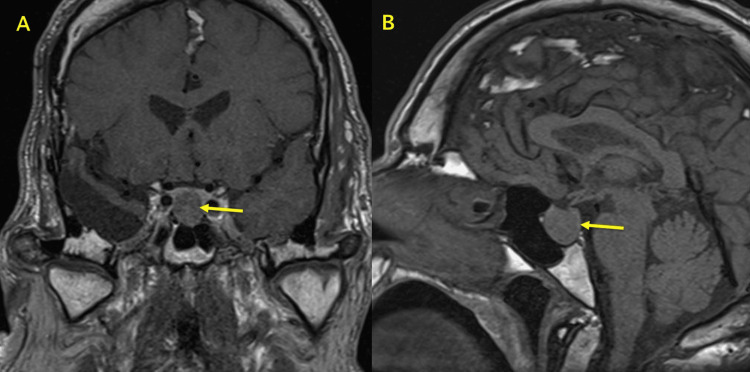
MRI showing pituitary adenoma Coronal (A) and sagittal (B) MRI images demonstrating a pituitary macroadenoma measuring approximately 17 mm craniocaudally and 16 mm anteroposteriorly. The lesion does not encase the carotid arteries, with preserved pituitary stalk position and a normal-appearing optic chiasm. A previous right temporal lobe lobectomy can also be seen in the sagittal (B) image
